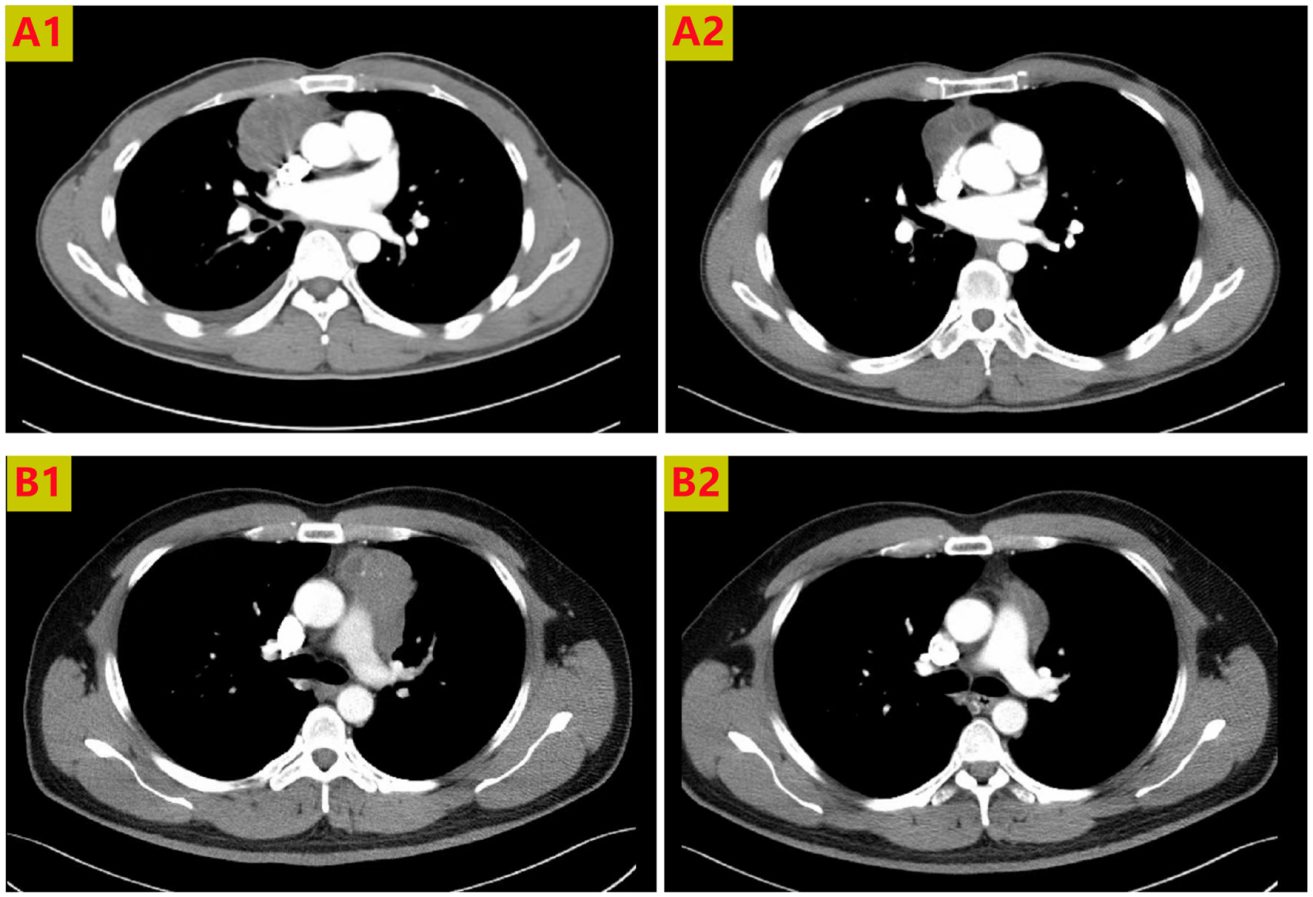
Chest CT scans of 2 patients receiving neoadjuvant radiotherapy. The anterior mediastinal lesion shrank more than two-fold in volume. Thoracic CT scans showing tumors before treatment (A1, B1), and partial response to radiotherapy (A2, B2).
